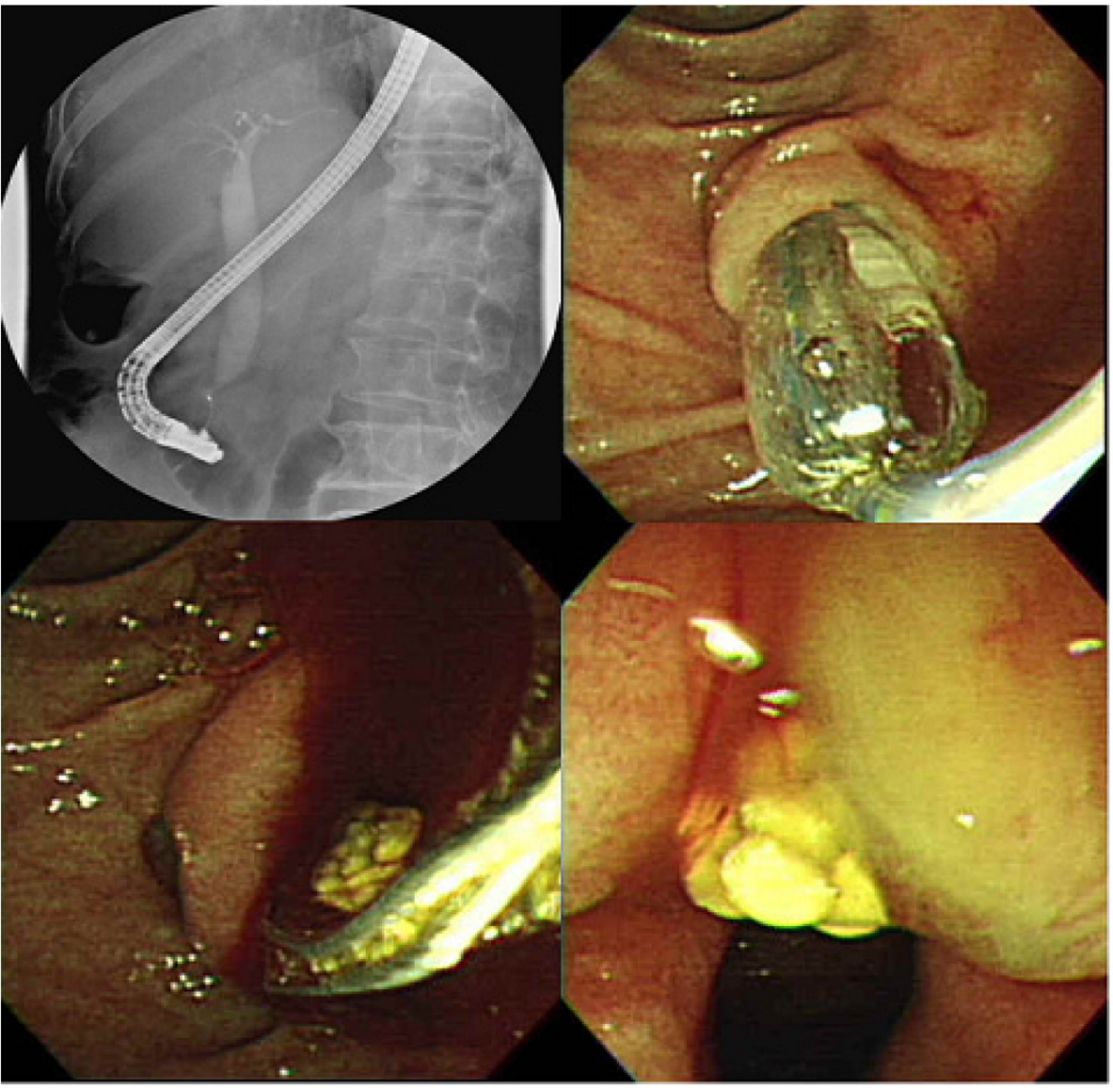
A female patient presented with epigastric pain, jaundice, while CT scan showing dilated CBD and suspicious of CBDS. In addition, no definite filling defect was found by ERC. However, EPBD was performed and a 1-cm hard stone was extracted subsequently
